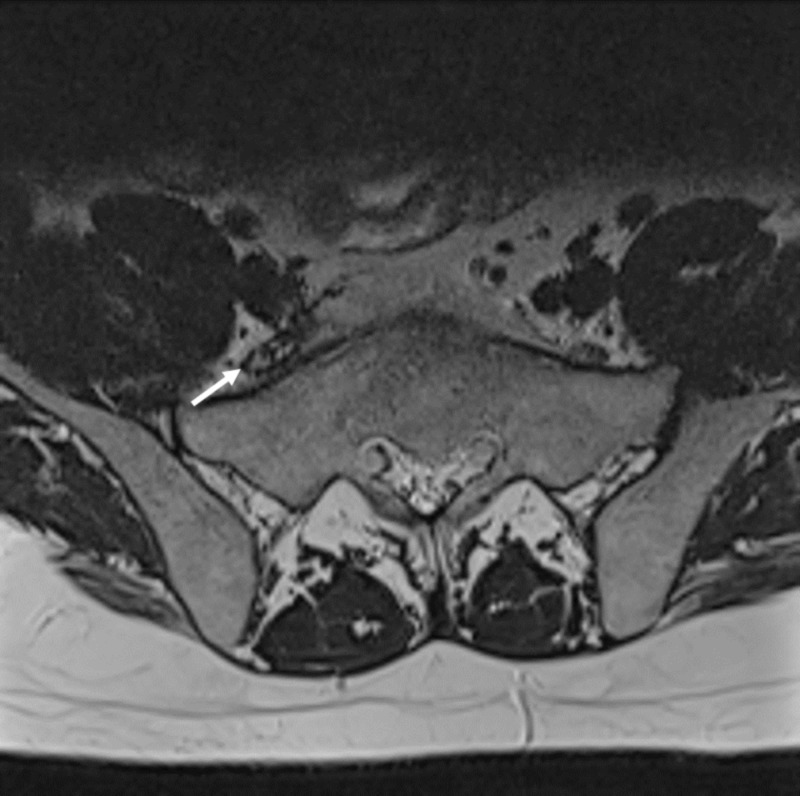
Post-operative magnetic resonance imaging Axial T2-weighted magnetic resonance imaging (MRI). Post-operative MRI showing adequate decompression and a hemosiderin rim surrounding the nerve (white arrow)
